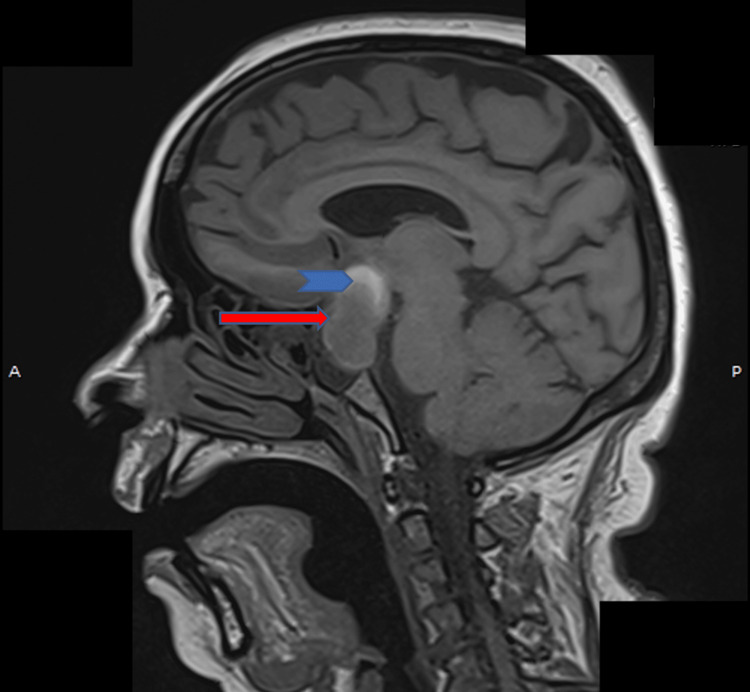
Sagittal T1-weighted MRI demonstrating a heterointense sellar-suprasellar mass with a characteristic “figure-of-eight” configuration (arrow) and hemorrhagic component in the superior portion of the lesion (arrowhead).
